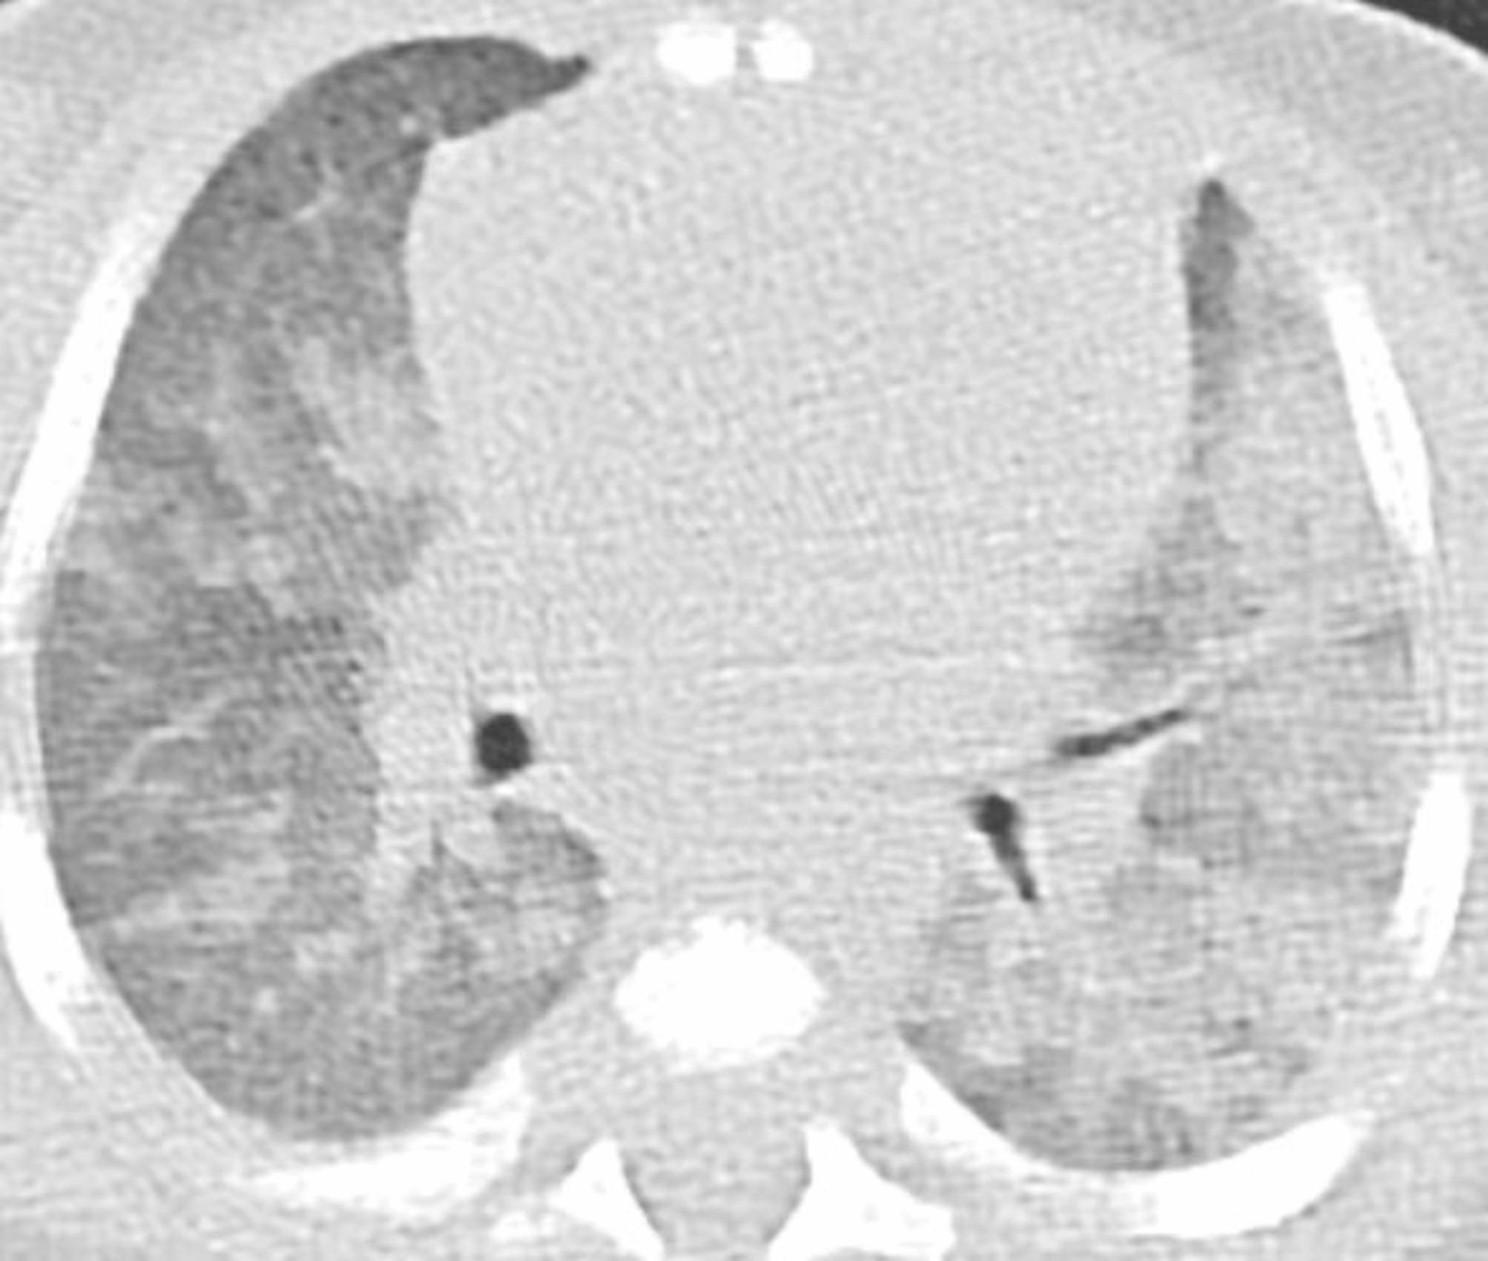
CT at the level of bronchial branches
